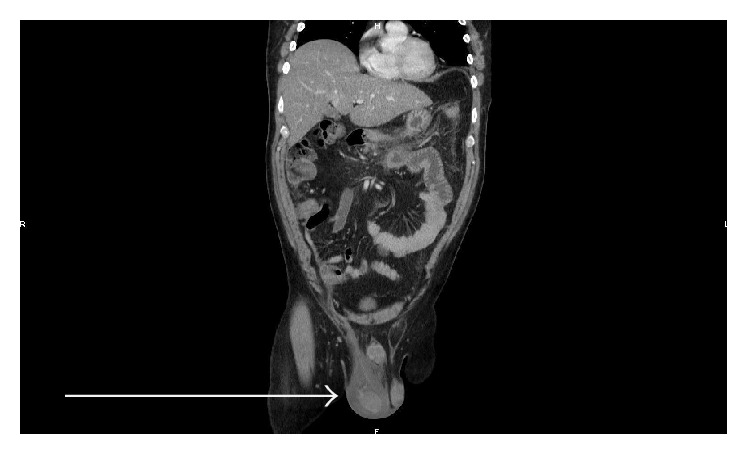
Frontal section contrast CT scan showing involvement of the right scrotum after 4 days of admission.
